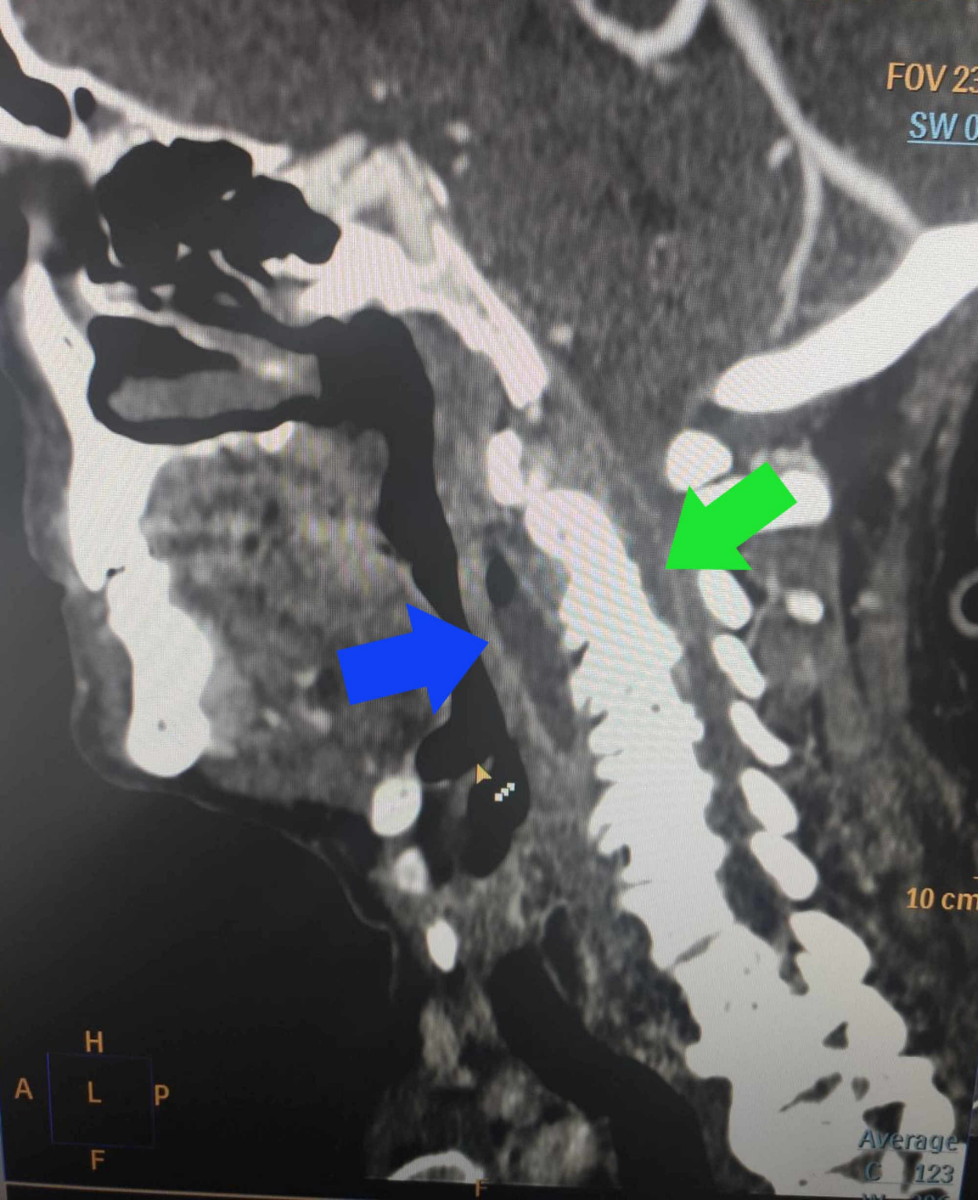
CT scan Contrast-enhanced computerized tomography scan with existence of retropharyngeal abscess (blue arrow) and epidural abscess (green arrow)
